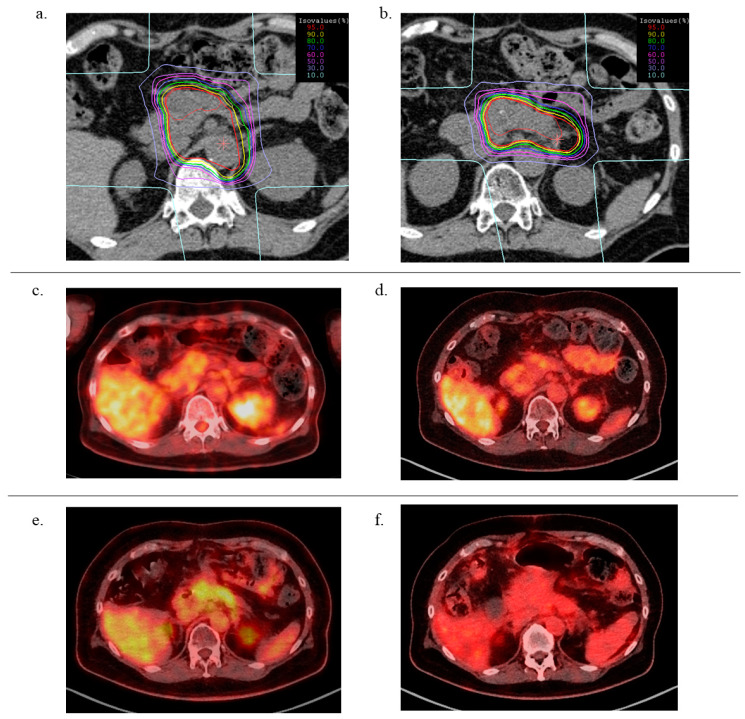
Typical case presentation: 55-year-old female patient with pancreatic body cancer. (a) Dose distribution of 1st CIRT, (b) dose distribution of 2nd CIRT, (c) fluorodeoxyglucose (FDG)-positron emission tomography (PET) computed tomography (FDG-PET/CT) image before 1st CIRT, (d): FDG-PET/CT image at 3 months after 1st CIRT, (e): FDG-PET/CT image at the recurrence after 1st CIRT, (f): FDG-PET/CT image at 3 months after 2nd CIRT.
